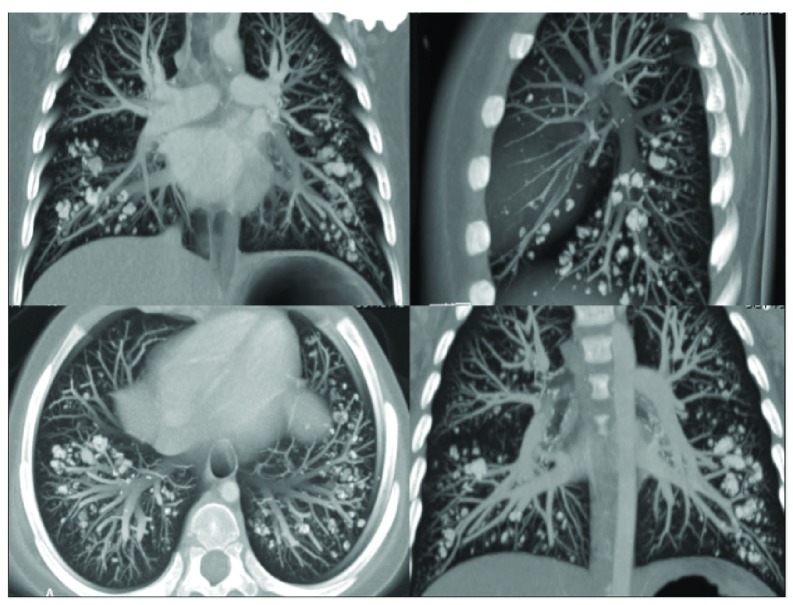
Thorax CT scan: pulmonary alveolar calcification.
